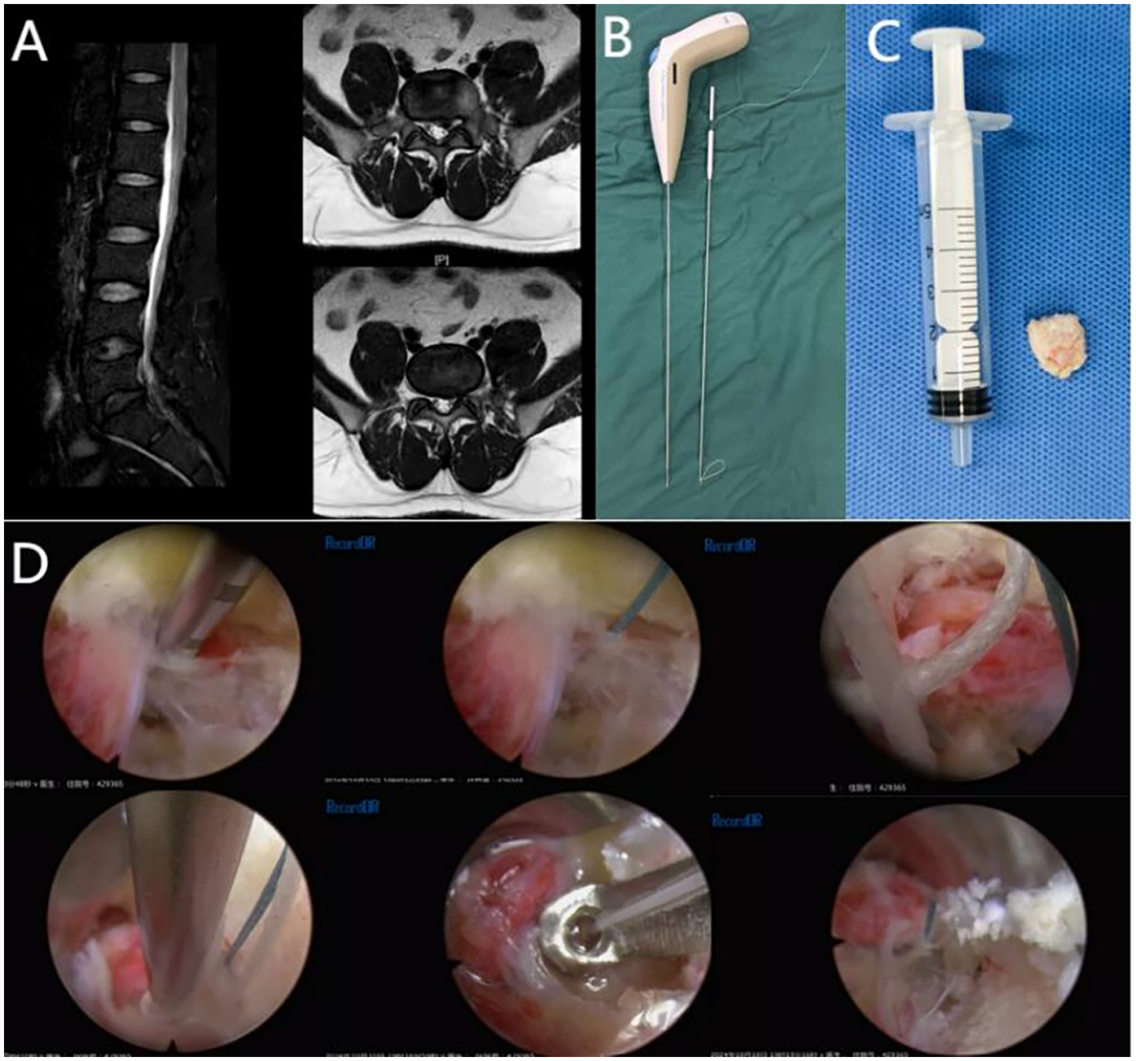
Preoperative MRI (L5-S1) and intraoperative data of the representative case are shown in the figures. (A) Preoperative MRI images of a 43-year-old obese patient with lumbar disc herniation (L5-S1). (B) The Disposable Annulus Stapler. (C) The nucleus pulposus removed from the patient during PTED. (D) Post-PTED double-stitch suture technique for annulus defect closure.
